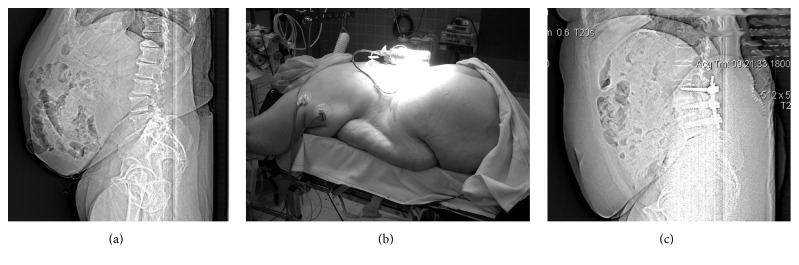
Example of obese patients with BMI > 30. (a) Presurgical CT scout of a 64 y.o. male patient with BMI 34.6. (b) Severely obese 59 y.o. female patient with BMI 37.1 positioned on the OR-table. (c) CT scout of the patient shown in (c) after two-level PLIF.
